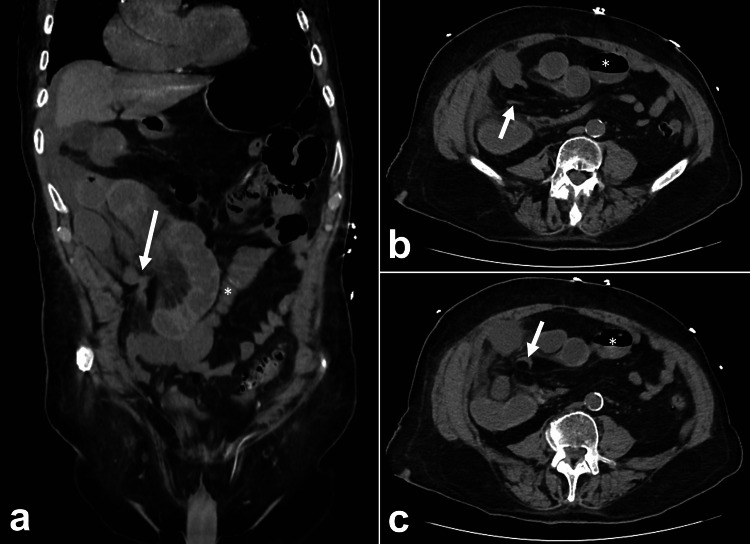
Computed tomography of the abdomen and pelvis with contrast showing multiple dilated, fluid-filled segments of the small bowel in the right hemiabdomen with surrounding ascites, intestinal edema, and mesenteric fat stranding. The cecum and ascending colon (asterisk) are displaced anteromedially (a) The coronal view shows the site of strangulation (arrow). The axial view shows the (b) proximal transition point (arrow) and (c) distal transition point (arrow) of the closed loop obstruction
